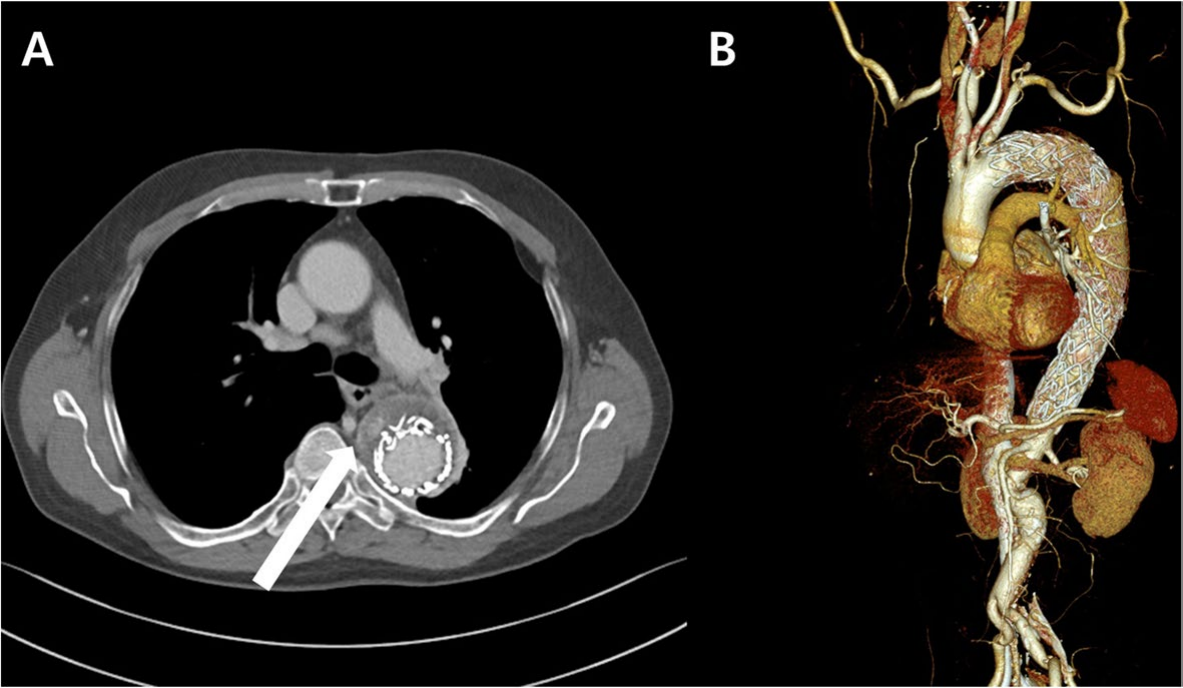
Preoperative CT angiogram. a Descending thoracic aortic aneurysm (maximal dimension of the aneurysm = 5.9 cm) with a type II endoleak (white arrow) in the delayed phase. b Post-TEVAR state showing multiple stent grafts from zone 3 to 5 of the aorta. CT: computed tomography; TEVAR: thoracic endovascular aortic repair
